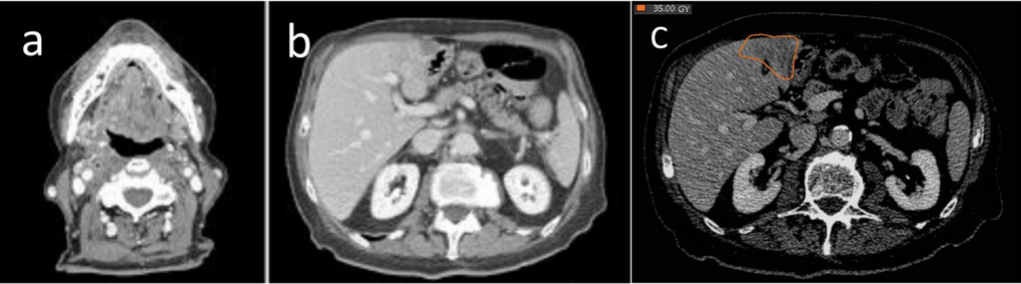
Constrast-enhanced total body CT scan after 2 months from the end of radiotherapy. Hypodense halo, corresponding to the prescription isodose (80%) at the first CT-scan evaluation 2 months after treatment.
